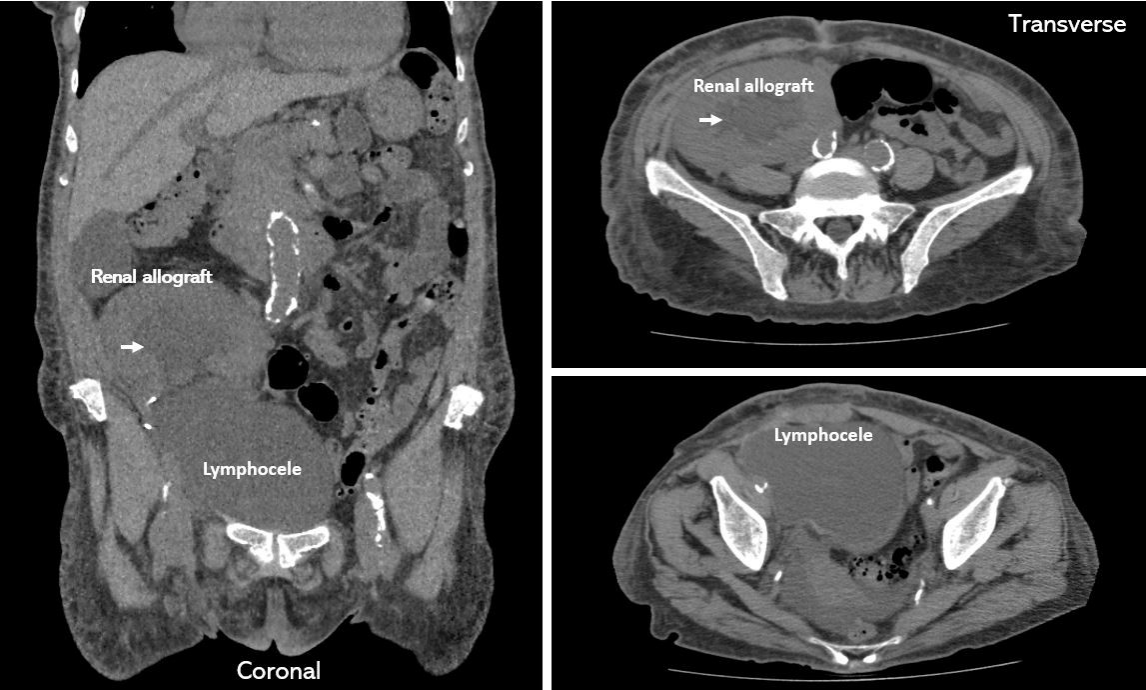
Computed tomography (CT) scan of the abdomen and pelvis without contrast demonstrating a large lymphocele occupying most of the pelvis. Arrows point to dilated renal collecting system/hydronephrosis.
